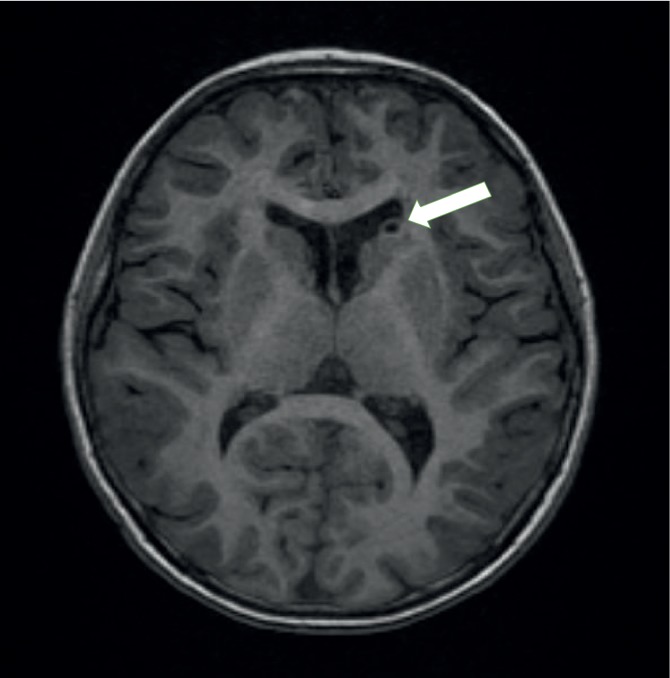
MRI finding of a subependymal nodule (SEN) (white arrow).
